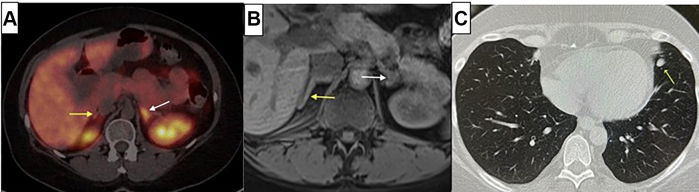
Multimodality imaging findings: (A) ^68^Ga-DOTATATE PET/CT revealed asymmetric focal radiotracer uptake in the *left* adrenal gland (standardized uptake value [SUV]max 17.3, Krenning score 3) (white arrow) compared to the *right* adrenal gland (SUVmax 9.0) (yellow arrow). (B) Abdominal magnetic resonance imaging demonstrated mild bilateral adrenal thickening (yellow and white arrows) with an 8 mm *left* adrenal nodule (white arrow) showing imaging characteristics consistent with a benign lipid-rich adenoma. (C) Chest CT from follow-up 18F-fluorodeoxyglucose PET/CT study identified an 8 mm lingular pulmonary nodule (white arrow).
